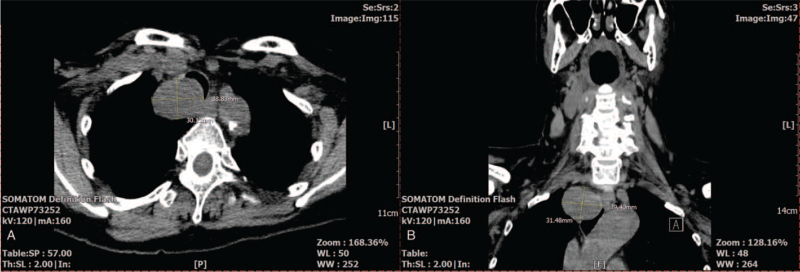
The tracheal mass compressing the airway shown in the axial (A) and coronal view (B) of the preoperative nonenhanced neck computed tomography.
